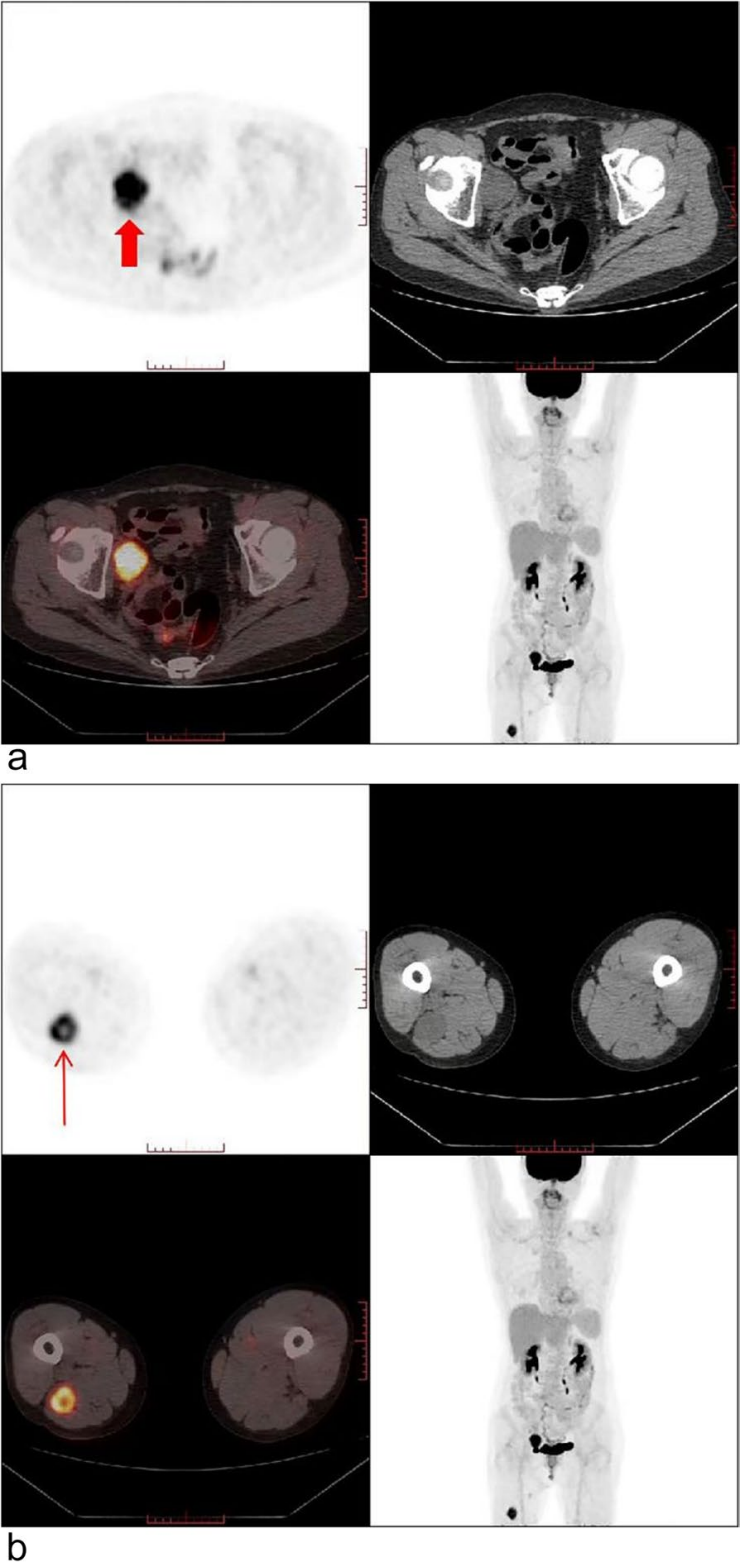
The trunk PET/CT examination confirm the presence of the pelvic mass and the lesion in the right thigh muscles. a and b Both the pelvic mass (thick arrow) and the mass in the right thigh (thin arrow) show high tracer uptake. *PET/CT *positron emission tomography and computed tomography
